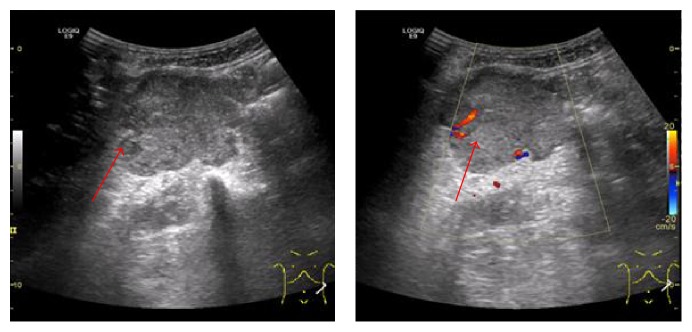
Ultrasound shows hypoechoic nodule in the hepatic lobe, and CDFI shows blood flow in the lesion. Lesion (red arrows) by liver puncture, which is guided by US, is proved to be PHC.
